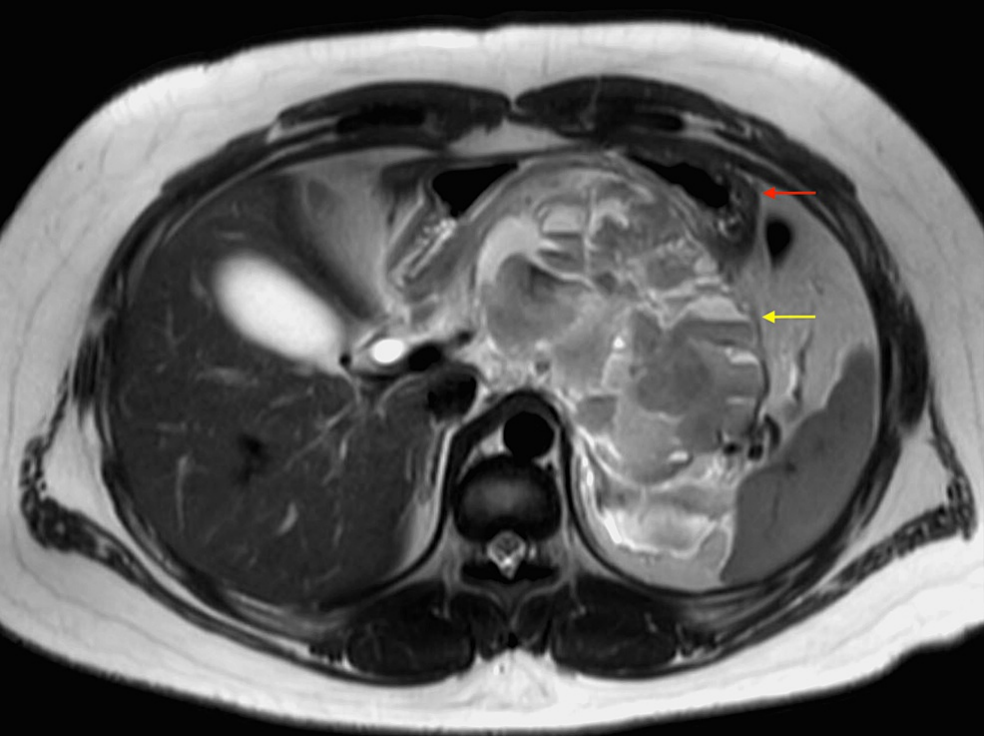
MRI of abdomen with lobulated intra-abdominal mass (yellow arrow) posterior to stomach (red arrow).
